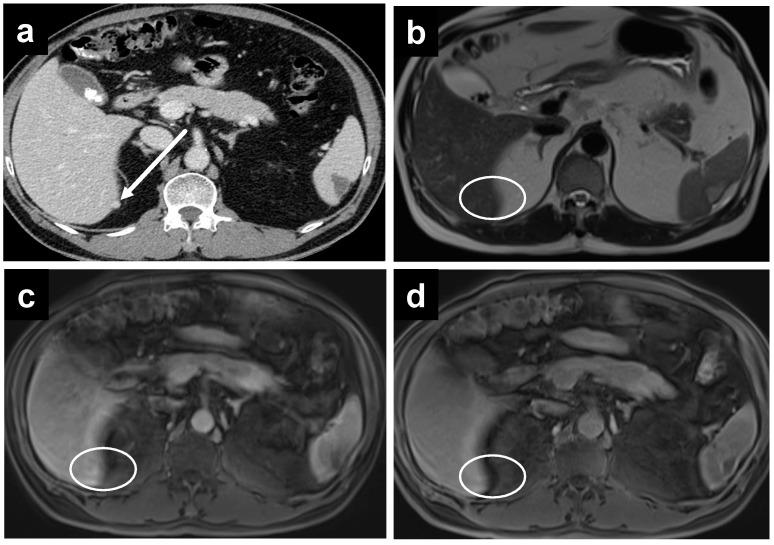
A 63-year-old male with malignant epithelioid mesothelioma. An enhancing perihepatic nodule is well demarcated on (a) CT but not visualized on (b) T2W, (c) portal venous or (d) delayed phases on MRI performed on the same day, due to motion artifacts.
